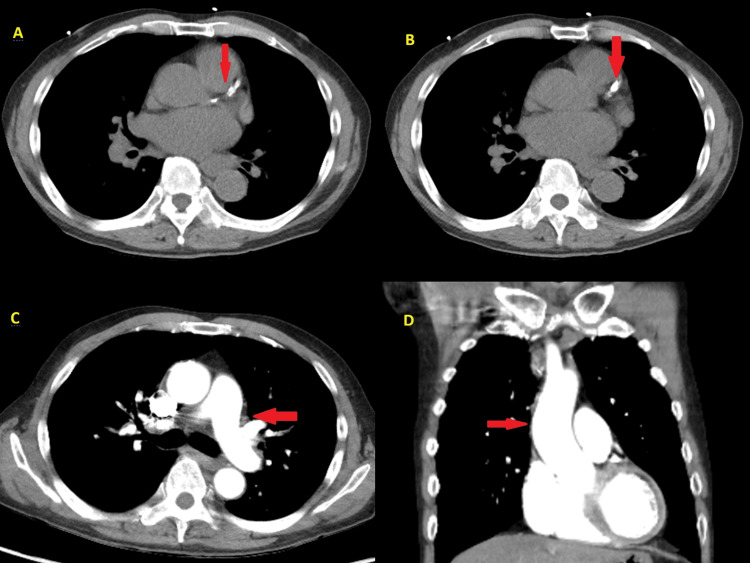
Contrast-enhanced chest CT (A and B) Axial views demonstrate significant coronary artery calcification (red arrows). (C and D) Visualization of the aortic arch confirms the absence of an intimal flap or aortic dissection. CT: computed tomography
